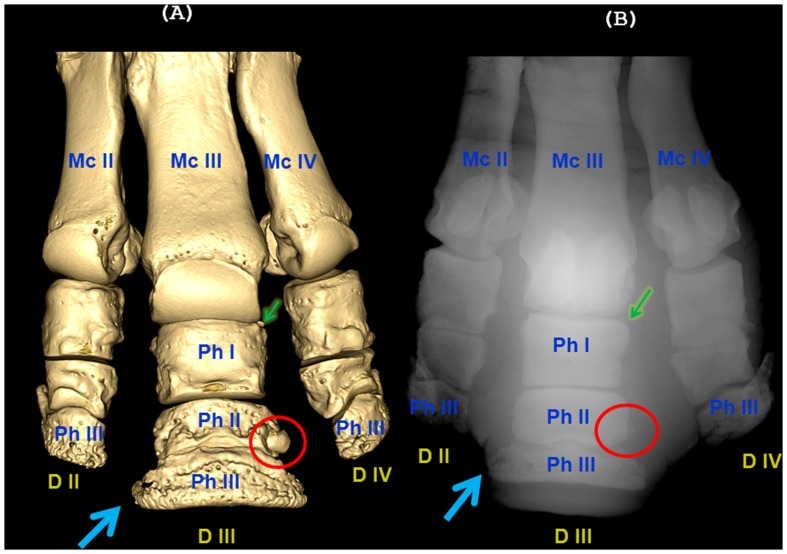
Bone pathology of the distal left front limb in rhinoceros 3. Computed tomographic tridimensional images of the distal autopodium, dorsal aspect (A) and the correspondent digital radiographic images, palmaro-dorsal view (B). The third or middle digit (D III) presents fractures on all three phalanges: the first phalanx (Ph I) - chip fracture with a small fragment (4.7 mm diameter) on the dorso-lateral aspect (A, B- small arrows); the second phalanx (Ph II) - dorso-lateral fracture with a displaced fragment (18,3×14,4×12,1 mm, A, B-circles); the third phalanx (Ph III) - chip fracture of the medial palmar process (processus palmaris medialis, A, B- large arrows).
